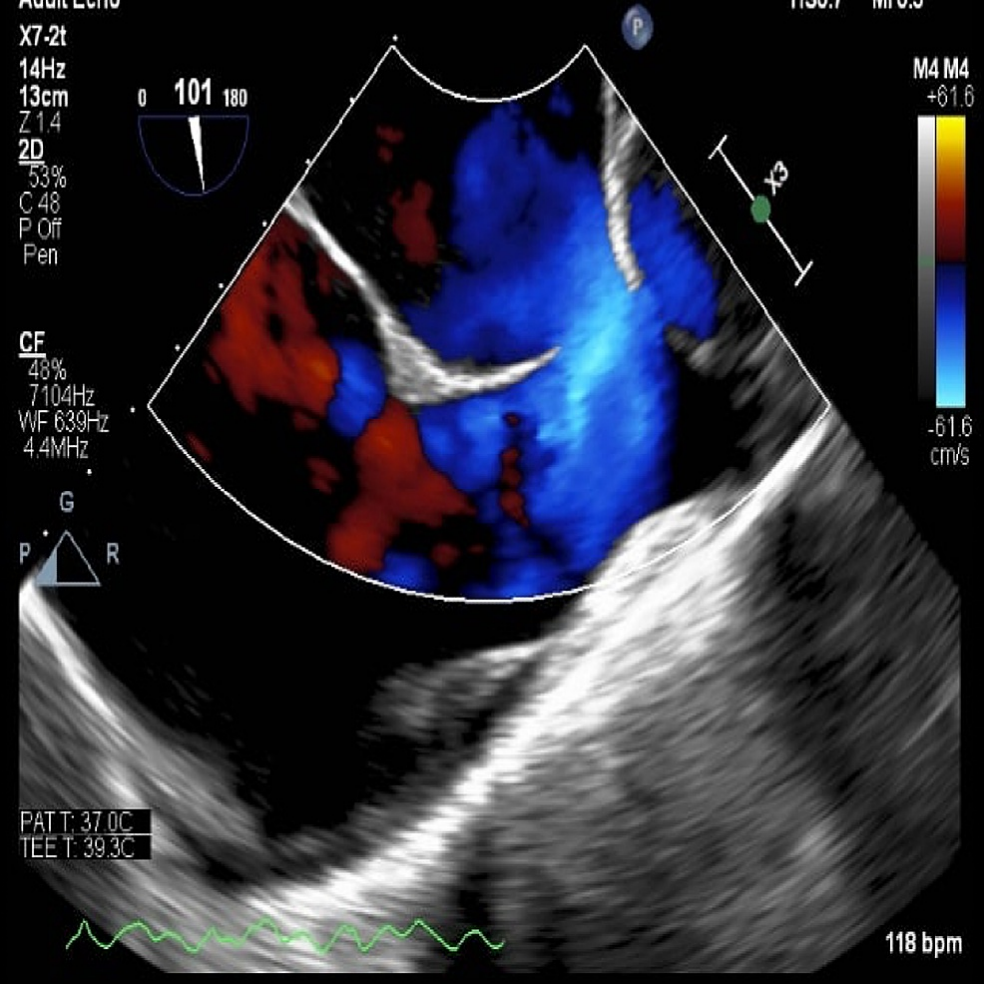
A color Doppler image from TEE shows a superior SVASD. TEE: transesophageal echocardiogram, SVASD: sinus venosus atrial septal defect.
